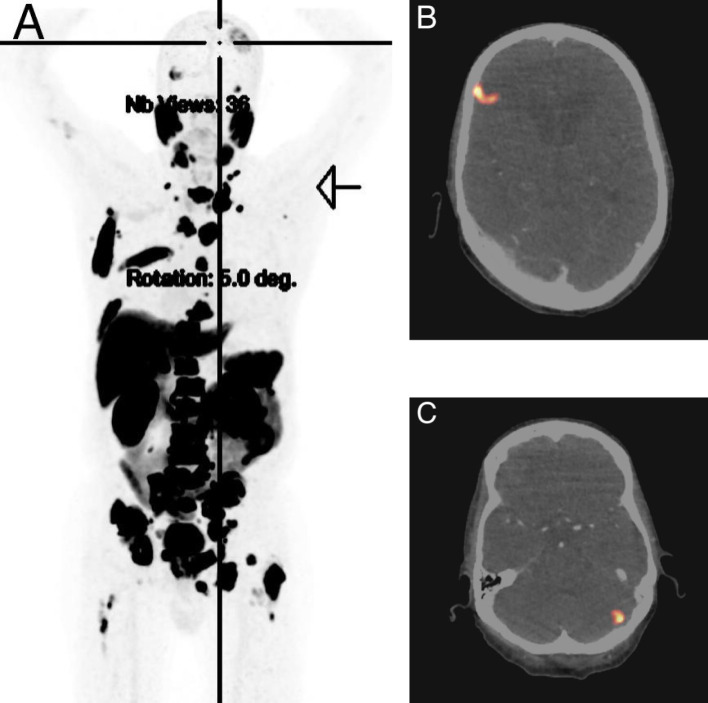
PSMA PET scout image (A) and PSMA avid right frontal (B) and left cerebellar (C) lesions.
